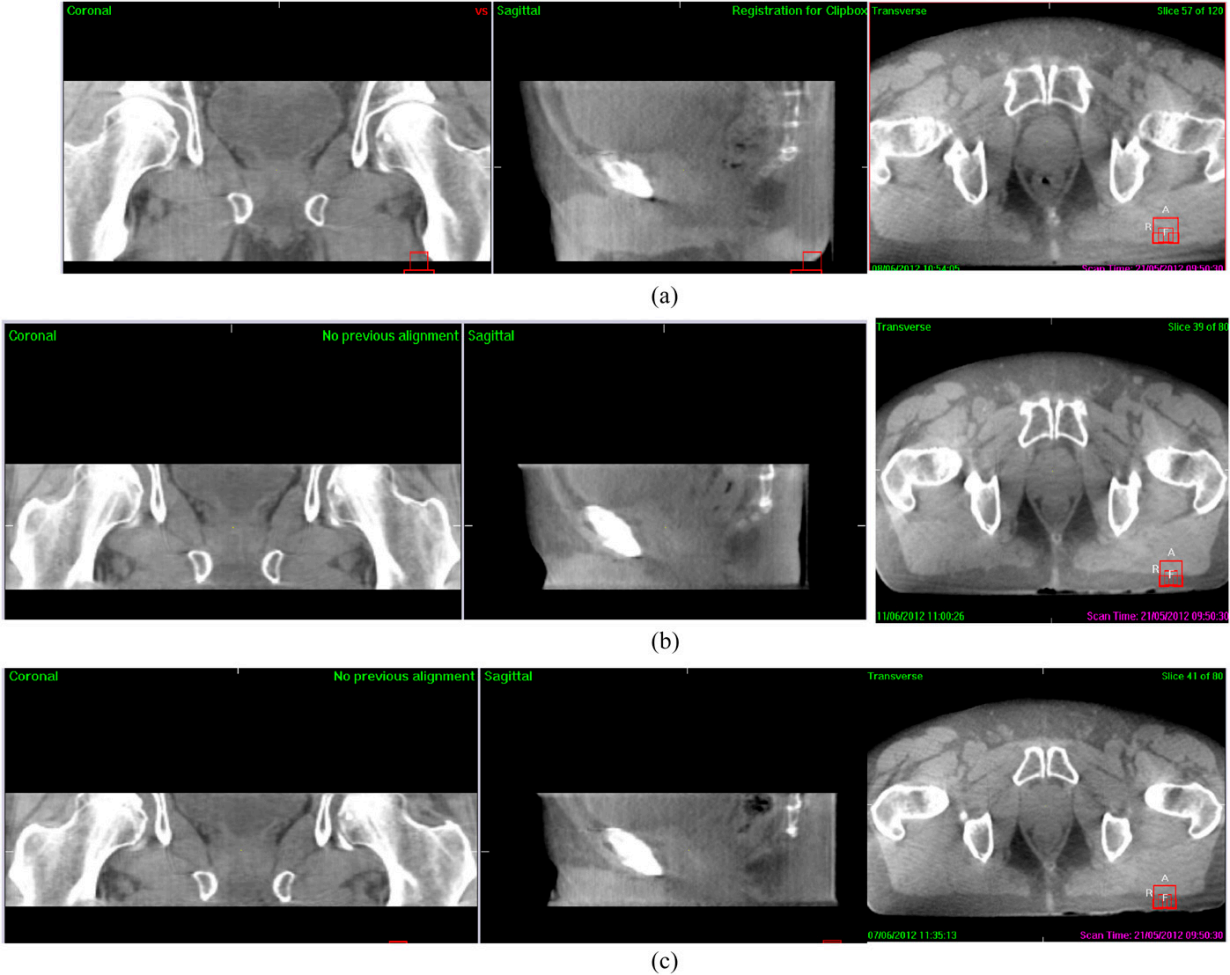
Cone beam CT acquired using (a) standard 13 cm length (M10; 1644 mAs), (b) 7 cm length standard dose (M5; 1644 mAs) and (c) 7 cm length high dose (M5; 2632 mAs).
